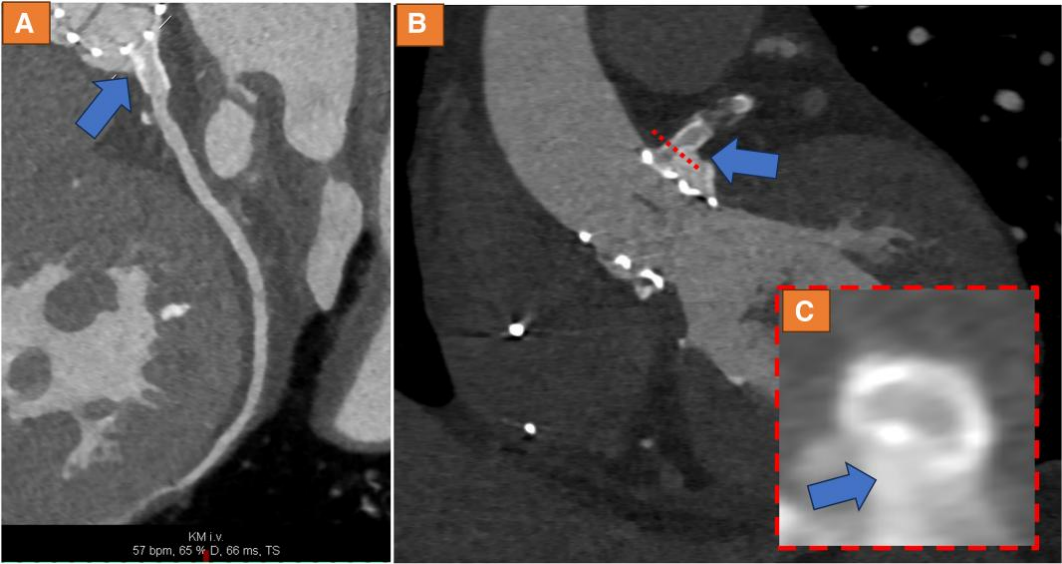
Cardiac computed tomography angiography after the procedure confirmed good lumen gain of the left main coronary artery (*A*), confirming the remaining external compression by valvular calcium (*B–C*).
